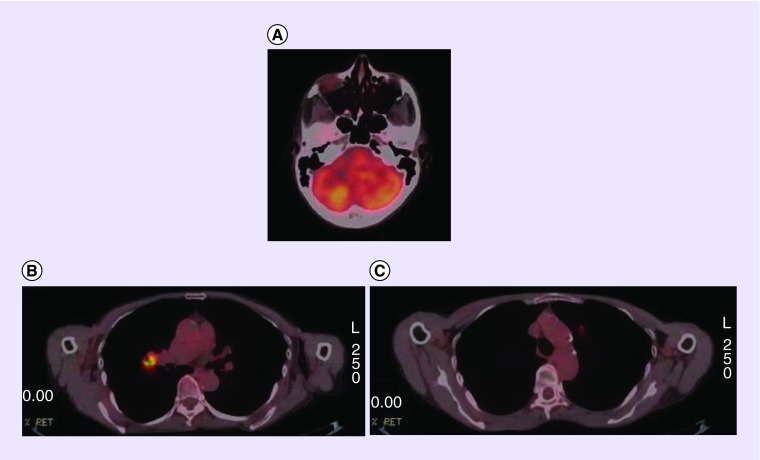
F-18 F-18 fluorodeoxyglucose PET/CT images of a patient with hypermetabolic brain lesion. (A) Axial projection of PET/CT images showing hypermetabolic brain metastasis in right cerebellar region. (B & C) Axial image of lung region showing right hilar hypermetabolic lesion and left metastatic pulmonary nodule.
